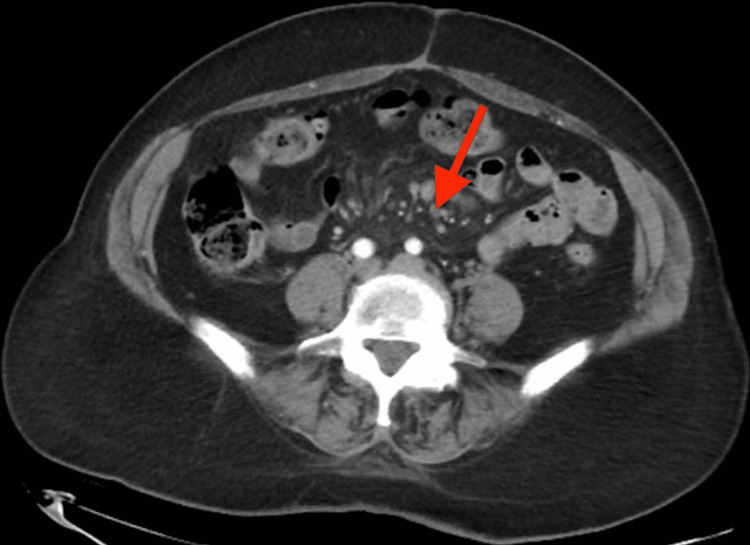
CT abdomen and pelvis with sub-centimeter lymph nodes and surrounding haziness and stranding in the root of the mesentery (red arrow)
